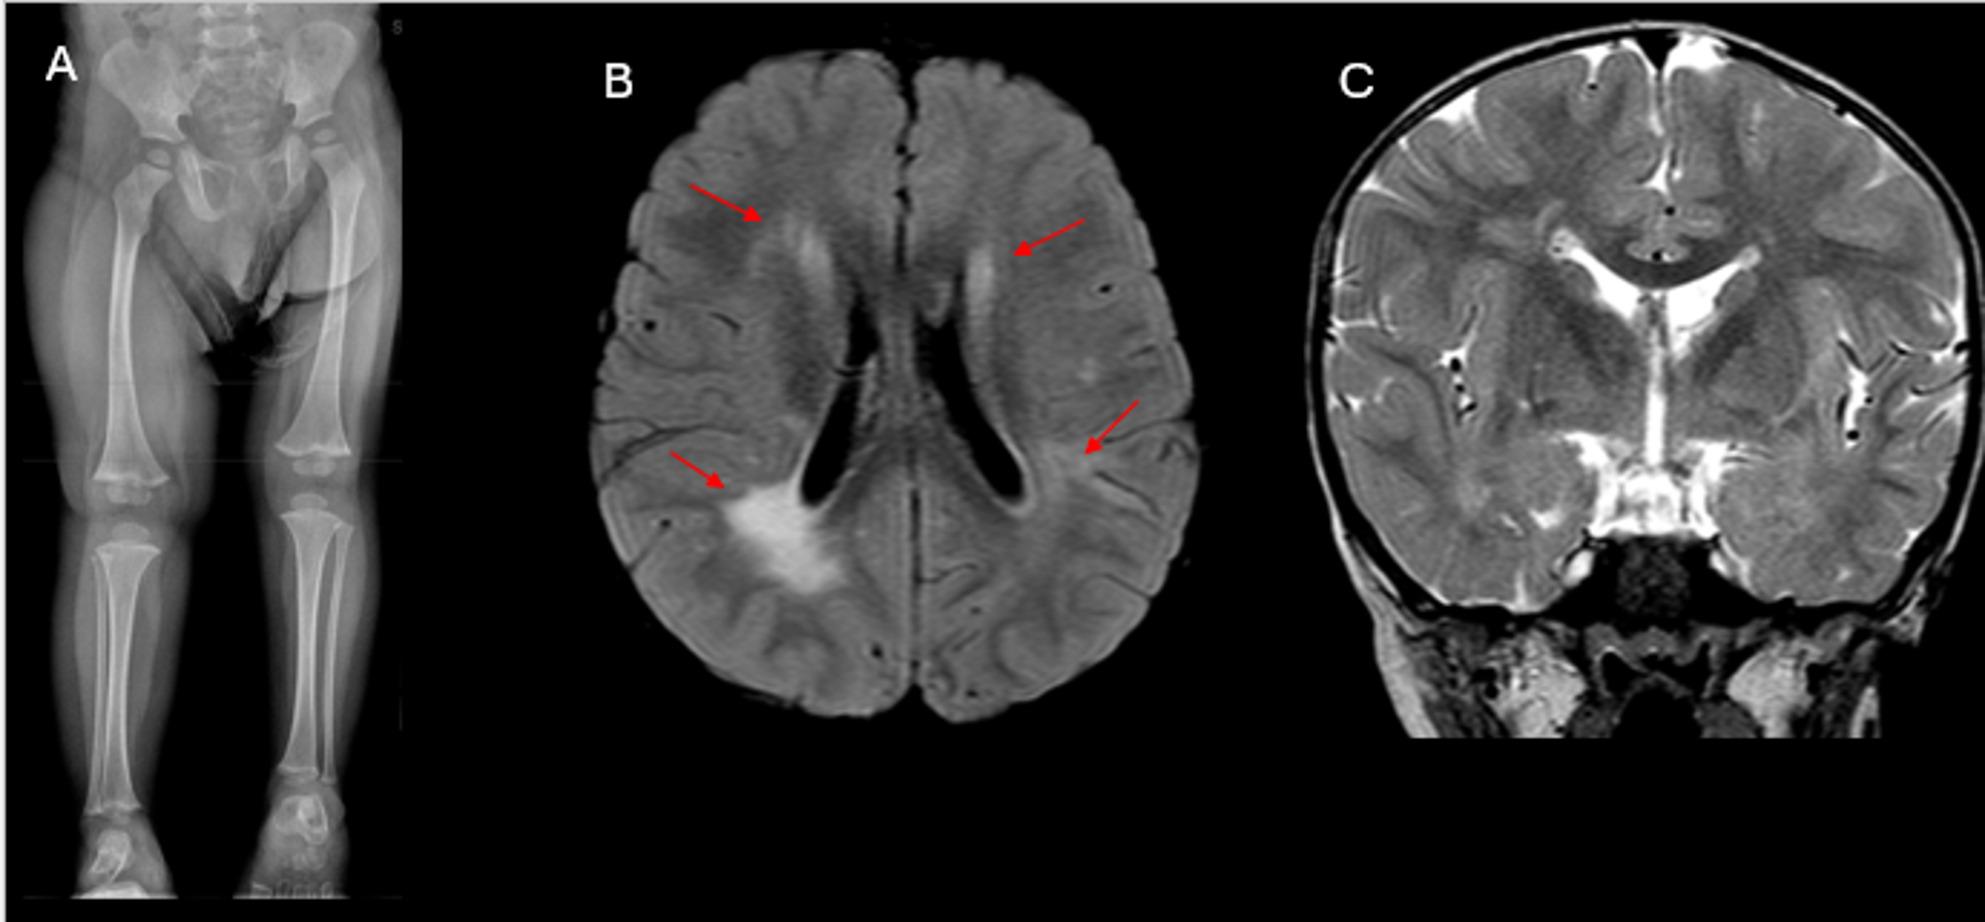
Child with Beckwith-Wiedemann syndrome. (A) Leg length radiograph demonstrates leg length discrepancy, with left lower extremity shorter than the right, and hypertrophy of the right lower extremity. (B) Axial FLAIR and (C) coronal T2-weighted images show asymmetric enlargement of the right cerebral hemisphere, consistent with right hemimegalencephaly. Additionally, abnormal T2 and FLAIR hyperintense gliosis is present in the periventricular white matter bilaterally (red arrows B)
